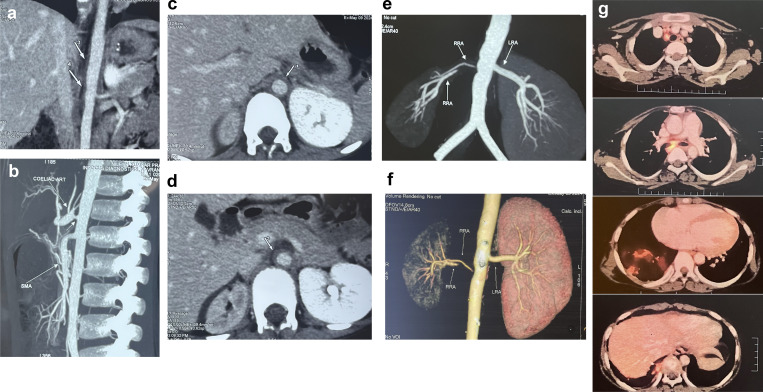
Panel of CT angiogram images showing vascular involvement and stenotic lesions and PET scan revealing non-necrotic lympadenopathy in patient 3. (a, c, and d) CT angiography of abdominal arteries of patient 3 shows concentric wall thickening from the level of the aortic hiatus, the entire suprarenal and renal segments (arrows labelled as 1 to 4 in a to d), and the short infrarenal segment of the abdominal aorta for a length of 9.0–9.5 cm and maximum wall thickness of 3.2 mm. (b–d) Mild eccentric narrowing at the celiac artery origin of about 20–30% stenosis, severe short-segmental stenosis/near-total occlusion at the origin of the anterior right renal artery (c), and long segmental occlusion for a length of 14 mm of the posterior right renal artery (d). The rest of the infrarenal abdominal aorta, aortic bifurcation, and both iliac arteries appear normal in course, caliber, outline, and branching pattern. (e and f) Right kidney (measures 7.5 cm) shows mild diffuse shrinkage in volume with mild hypoperfusion of the right kidney with delayed excretion, whereas the left kidney (measures 11.1 cm) shows compensatory enlargement, and normal perfusion and normal excretion. (g) Positron emission tomography scan shows no evidence of abnormal FDG uptake at concentric wall thickening/stenosis involving abdominal aorta and its branches. Multiple FDG avid patchy consolidation changes are seen mainly in the subpleural and few in the peribronchial regions of the lower aspect of the right middle lobe, and all basal segments of the right lower lobe. FDG avid small to mildly enlarged non-necrotic nodes are seen at right paratracheal, pretracheal, precarinal, subcarinal, right hilar, and right inferior pulmonary ligament, and lower paraesophageal regions may be noted.
